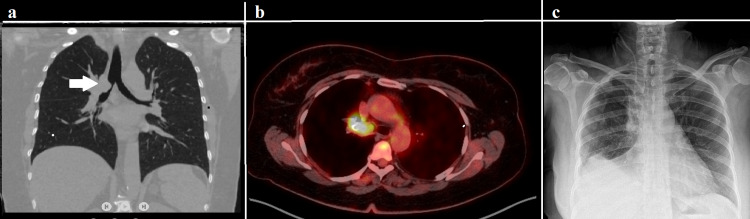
The chest CT showing a mass in the right upper lobe bronchus extending to the right main bronchus (arrow) (a); PET-CT showing a mass with high metabolic activity (b); x-ray, taken at discharge, showing the expansion of both lungs (c).
